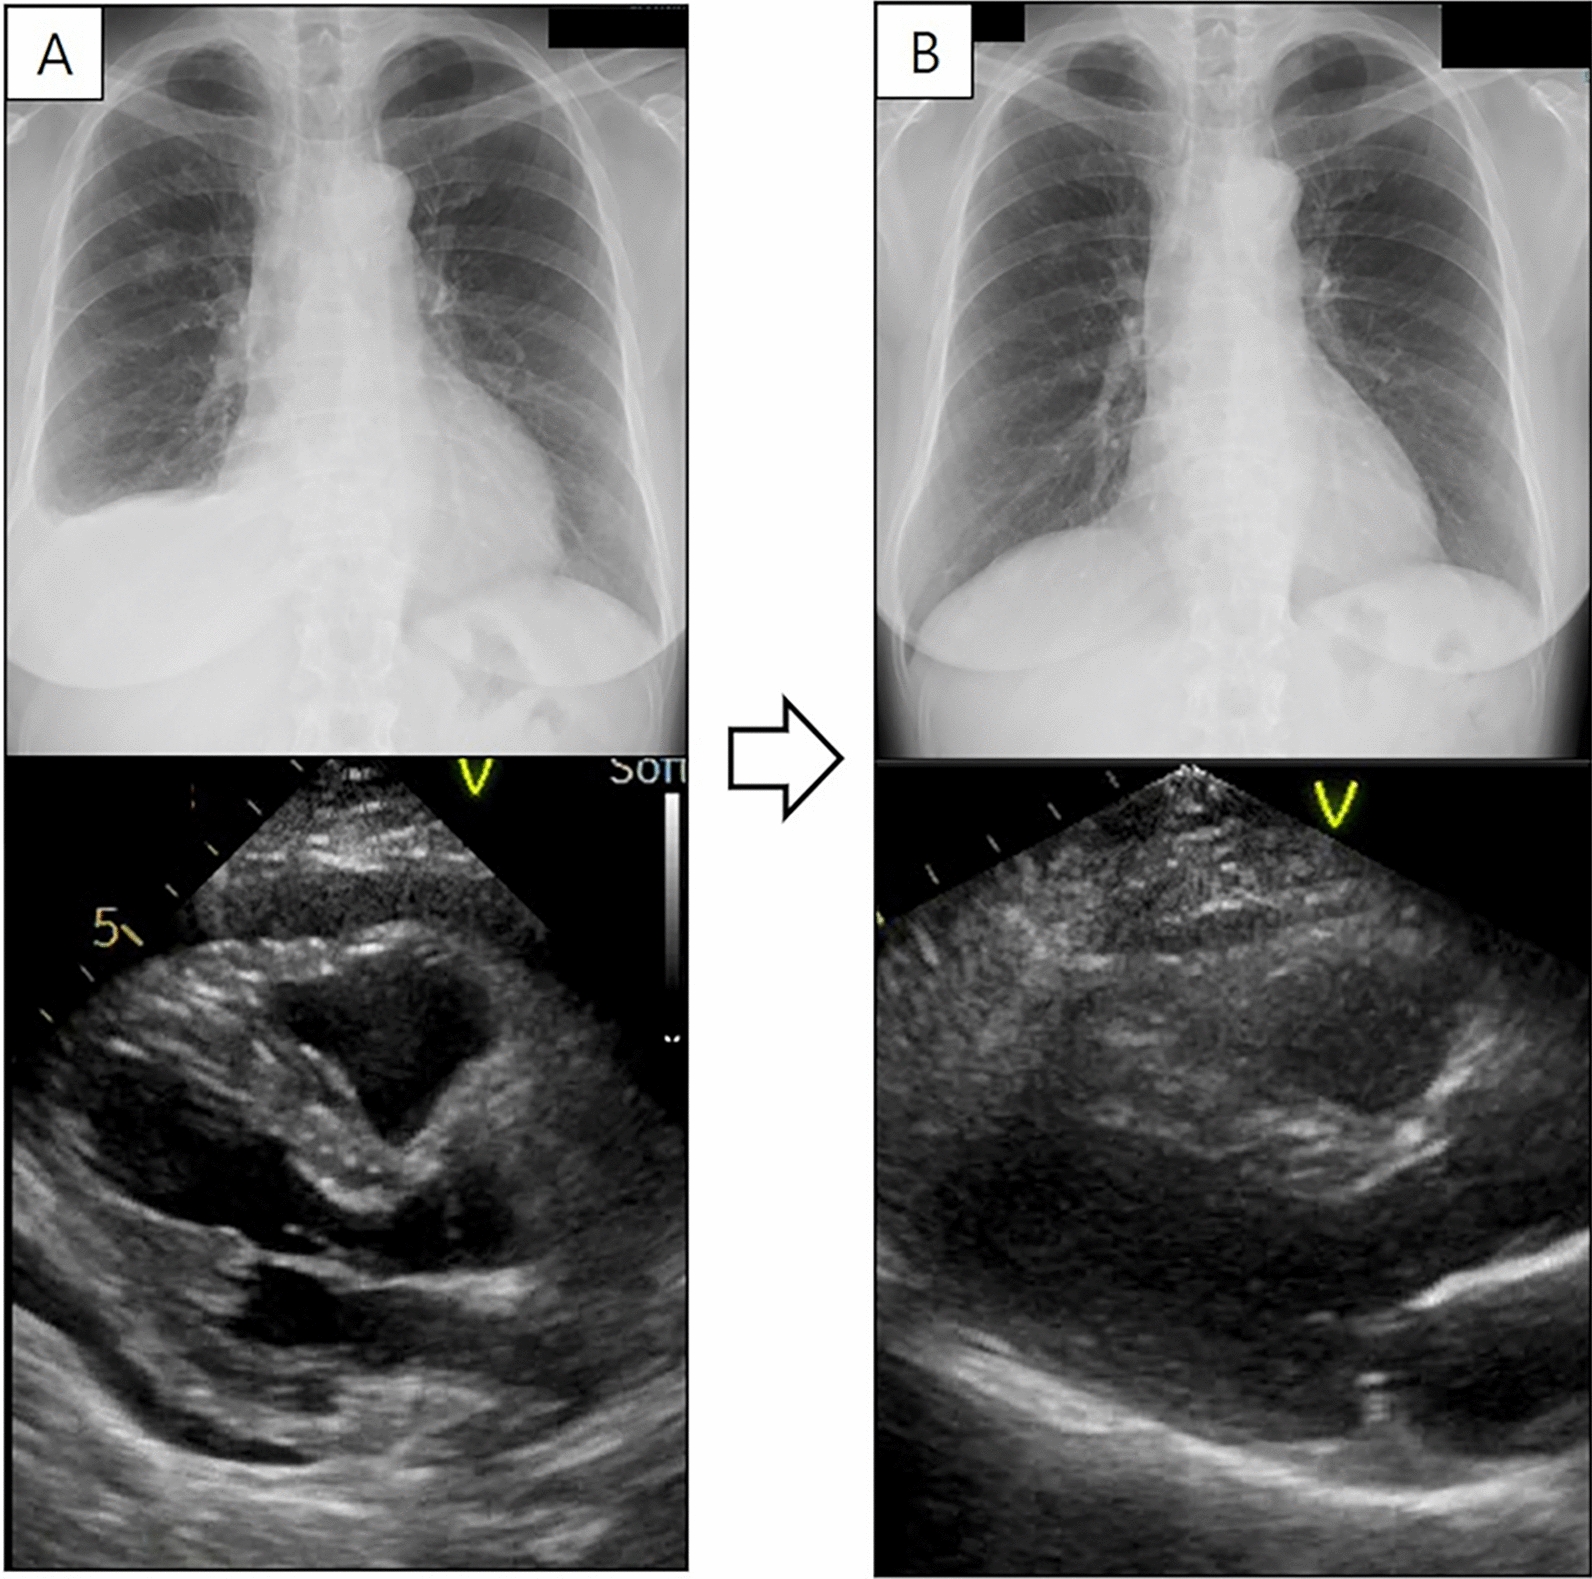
Compared with the pre-treatment (A), chest radiograph and cardiac echocardiography showed the pleural and pericardial effusions also completely resolved after antituberculous treatment (B)
